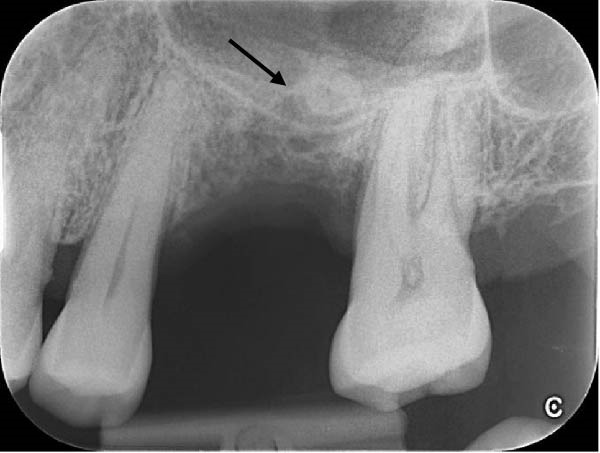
Pre‑operative periapical radiograph showing the distobuccal root fragment in the maxillary sinus region (arrow), which remained in the same position several weeks after displacement.
